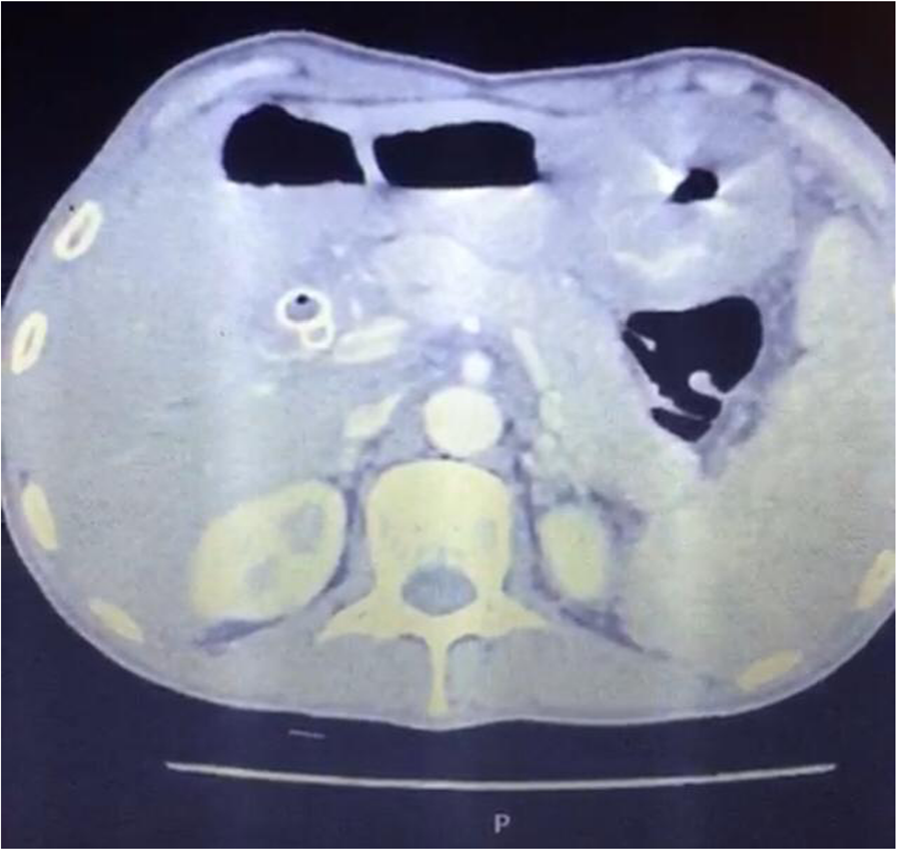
Abdominal CT revealing malignant gastric tumor
